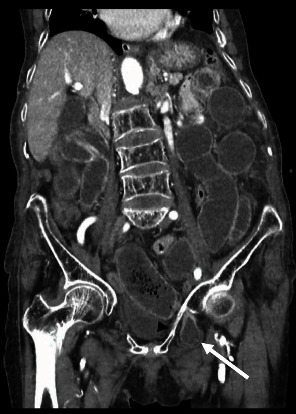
Coronal reconstruction of contrast-enchanted computed tomography: representative image showing a segment of small bowel protruding (white arrow) underneath the superior pubic ramus (black arrowhead) consistent with an obturator hernia. The rest of the small bowel is seen dilated up to 40 mm.
